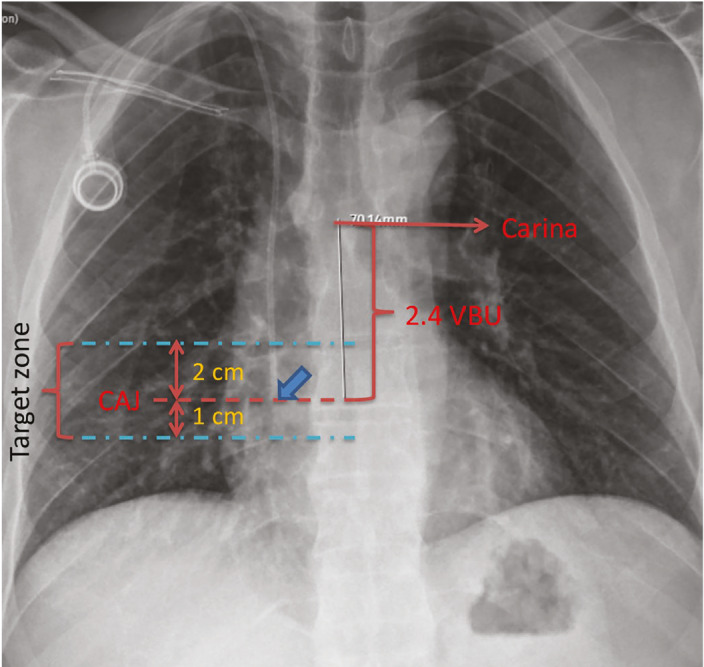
Measurements on chest radiography used to determine catheter tip position. The figure shows a totally implantable venous access port catheter tip positioned exactly at the CAJ. Blue arrow indicates position of catheter tip. CAJ, cavoatrial junction; VBU, vertebral body units.
